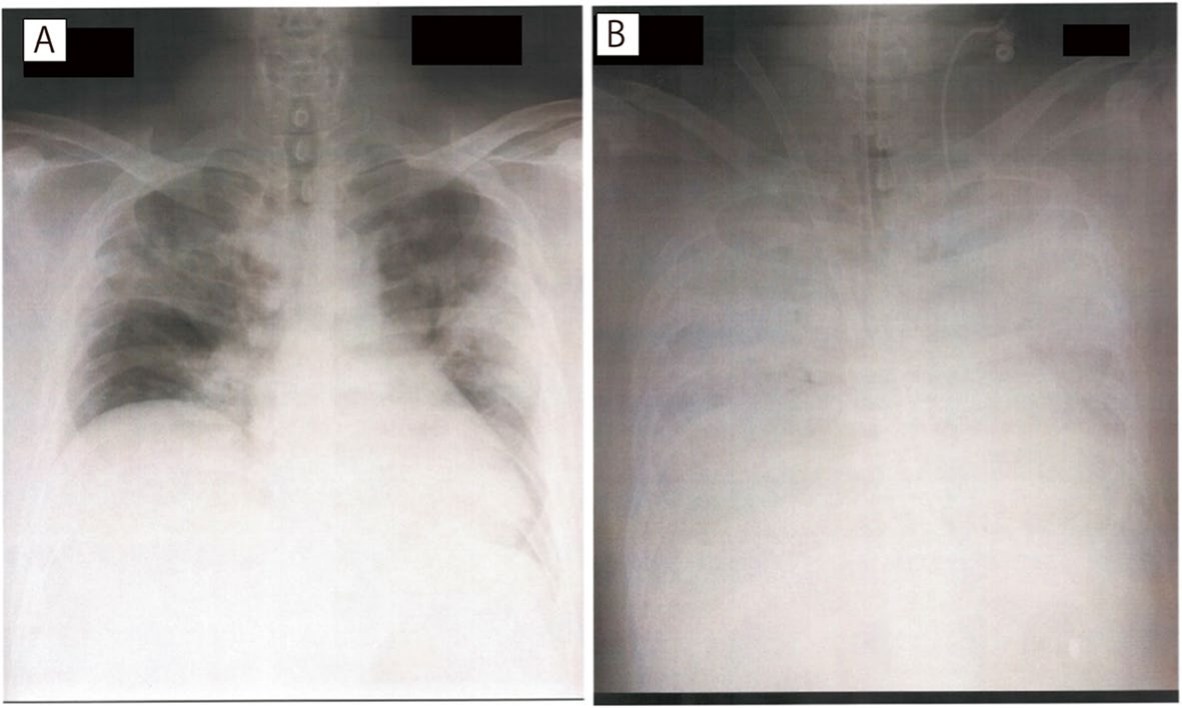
Chest radiograph on admission to the ICU (A) and immediately following the initiation of extracorporeal membrane oxygenation (ECMO) (B). Diffuse shadows in the bilateral peripheral lung on admission and enhanced pulmonary vascular shadow with cardiac enlargement and decreased permeability after starting ECMO should be noted
